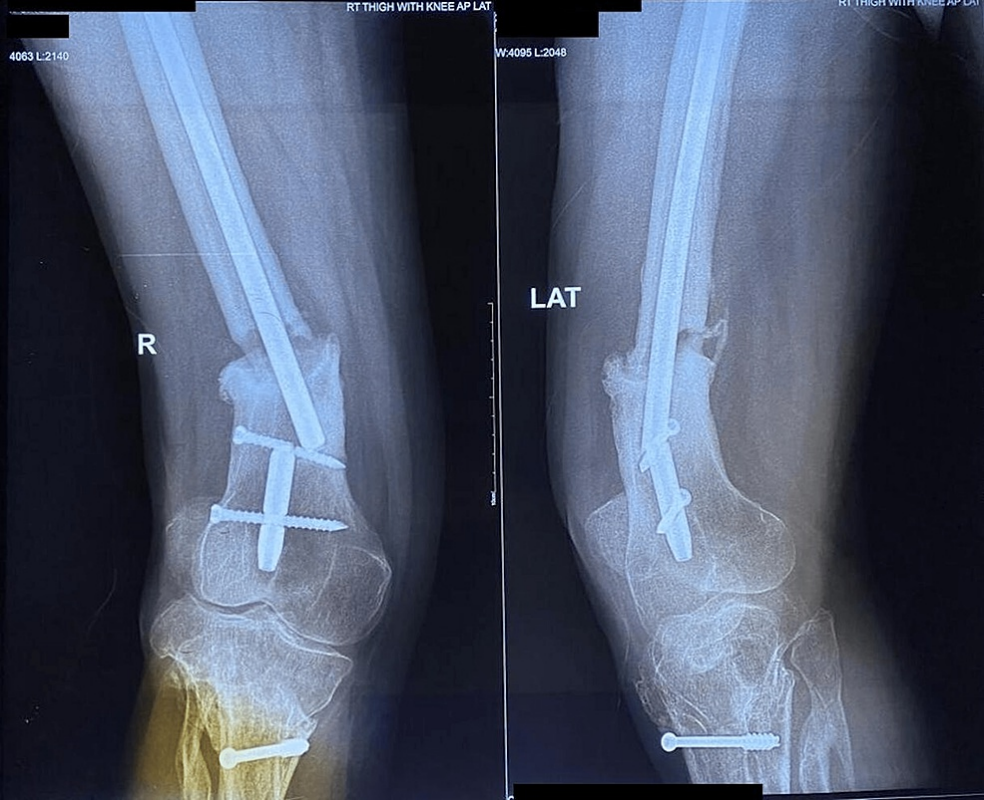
Pre-operative radiograph of patient 1 with broken intramedullary nail in situ.
